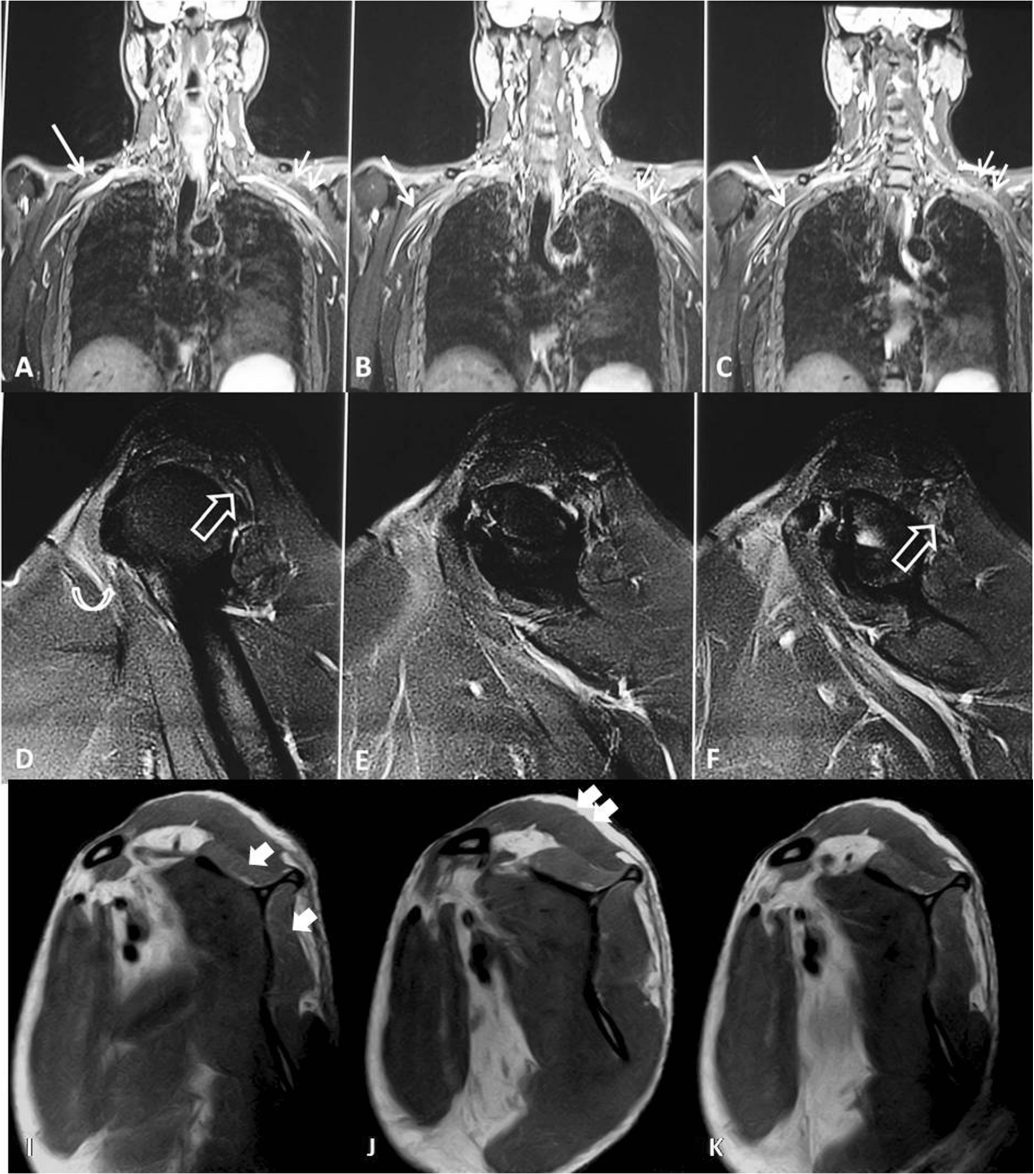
Magnetic resonance imaging of patient 2 depicting brachial plexus as well as periscapular muscles. (A,B,C) Coronal T2W fat suppressed contiguous section for brachial plexus shows edema in the trunks and cords of the right brachial plexus (straight arrow). Compare this to the normal brachial plexus on the left side (double arrow). (D,E,F) Sagittal T2-weighted fat-suppressed contiguous section at the level of the shoulder show edema and atrophy in the infraspinatus muscle (hollow arrow). Edema is also noted in the anterior fibres of the subscapularis muscle (curved arrow). (I,J,K) Comparable T1W sagittal images show fatty infiltration in the supraspinatus and infraspinatus muscles (solid arrows in I). Compare the increased intensity of these muscles as compared to the deltoid (double solid arrow in J), due to fatty infiltration.
